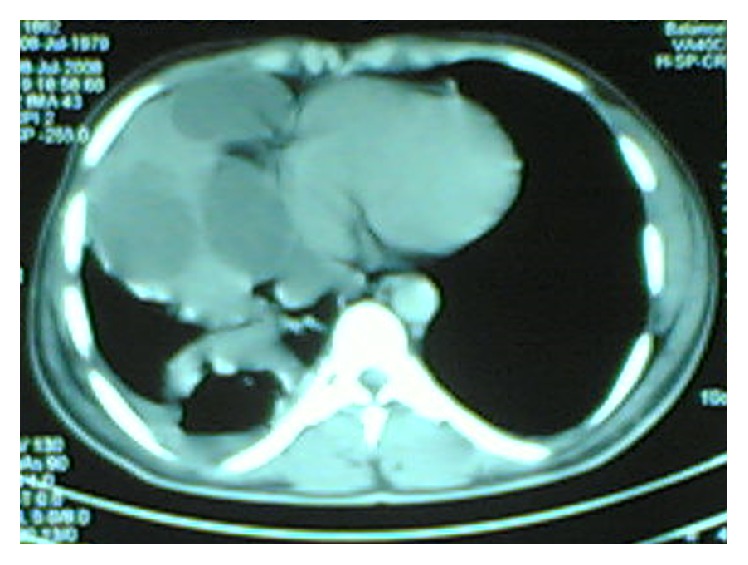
CT chest showing multiple large pulmonary hydatid cysts.
